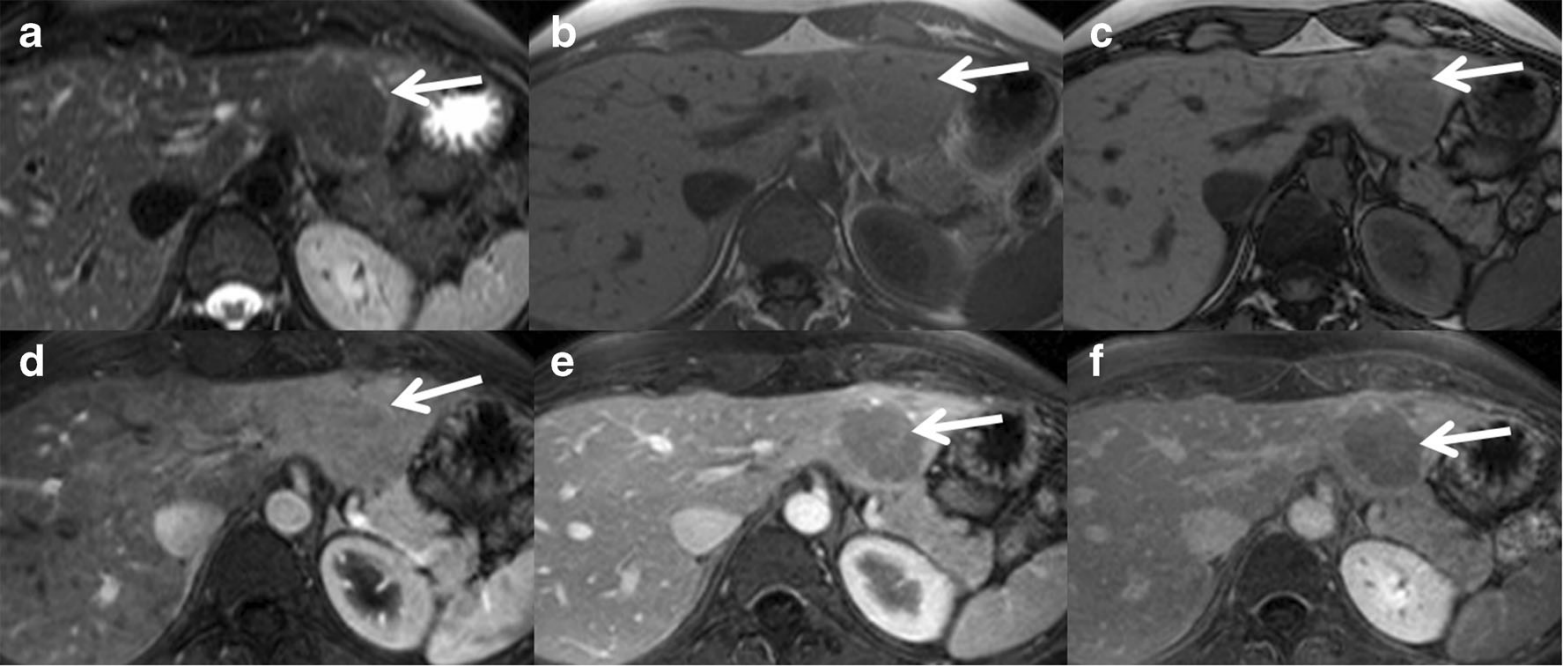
A 26-year-old female with right upper quadrant pain was found to have an echogenic nodule on ultrasound. (a) Axial T2W MRI shows an isointense lesion in the left lobe of the liver (*arrow*). Axial T1 in-phase (b) and out-of-phase (c) MRIs show a drop in signal in the out-of-phase images representing intralesional lipids (*arrow*). Axial post-contrast MRI in the arterial phase (d) shows mild diffuse enhancement of the lesion (*arrow*), not persisting in the portal venous (e) and delayed phases (f). MRI features are consistent with HNF-1α-mutated hepatocellular adenoma and the patient is on regular follow-up
